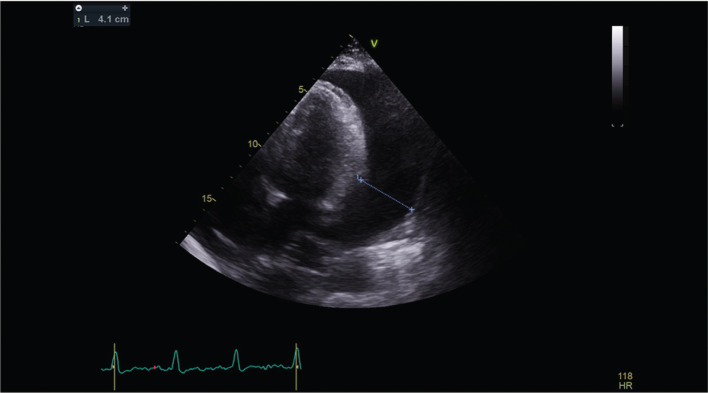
Large 4.1 cm anteroposterior diameter of a posterior pericardial effusion in an apical four-chamber view.
